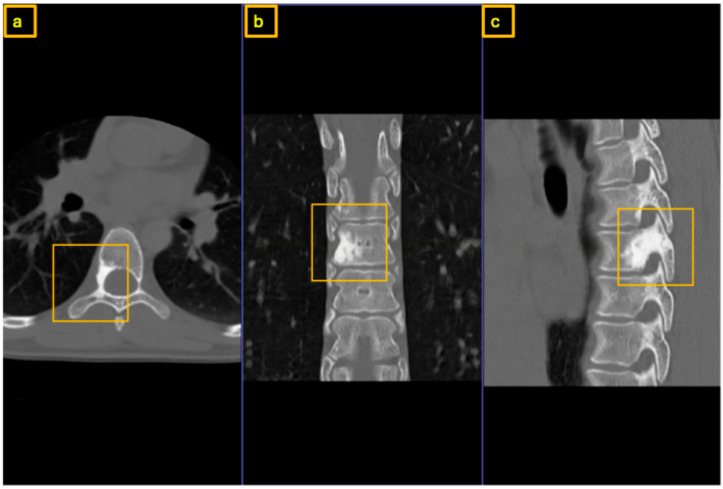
Preoperative CT of the thoracic spine for surgical planning: (a) axial view, (b) coronal view, and (c) sagittal view. The yellow square highlights the extent of the nidus within the posterior column, involving only the root of the right T7 pedicle.
